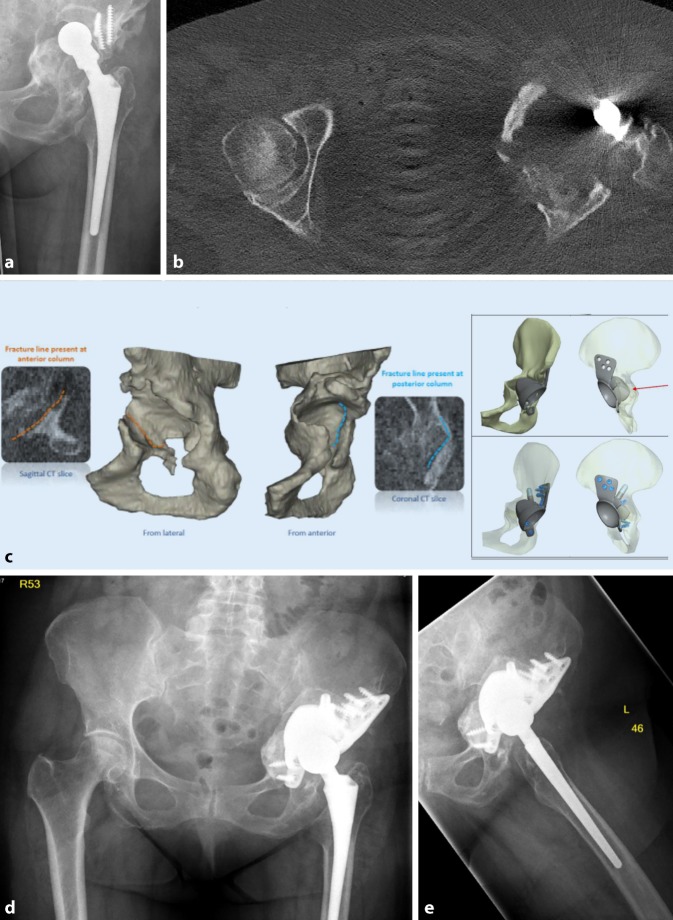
AP X‑ray of the hip (a) and CT scan (b) of a 77-year-old male patient with cup loosening, type IV acetabular bone defect, c imaging show preoperative planning. X‑ray of the hip AP and axial (d, e) after treatment with partial pelvic replacement (AQ Implants). (Courtesy of the Department of Diagnostic and Interventional Radiology, University Hospital of Leipzig, all rights reserved)
